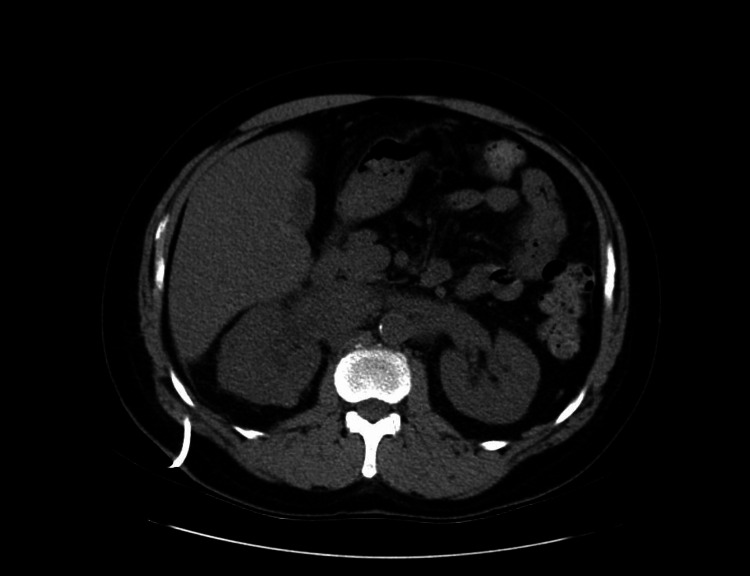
A Computed Tomography (CT) Scan of the Abdomen and Pelvis Revealed a Large Retroperitoneal Mass on the Right Side.
